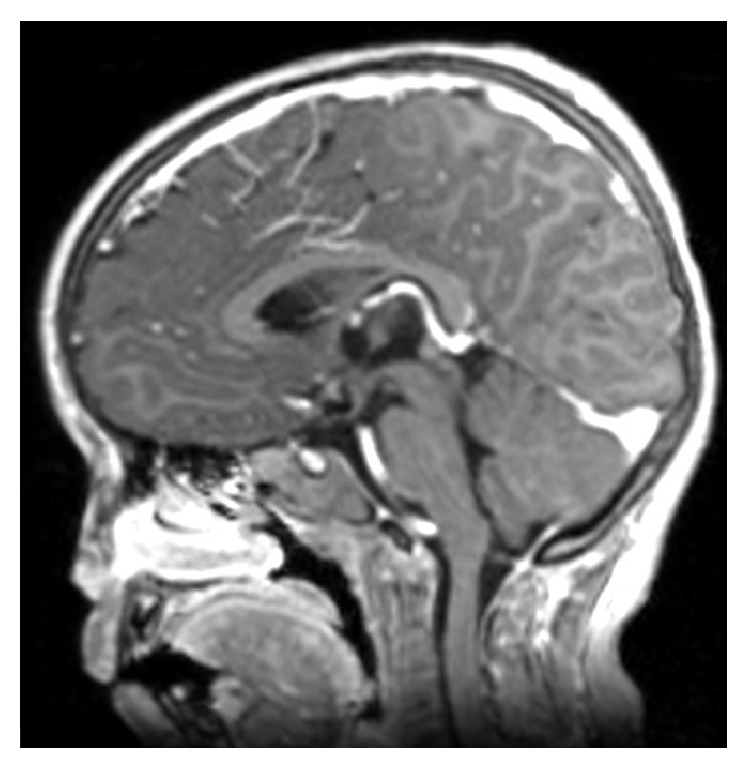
Follow-up image. Cerebral Magnetic Resonance Imaging enhanced with gadolinium contrast 22 months after intracavitary irradiation with radioisotope ^32^P.
